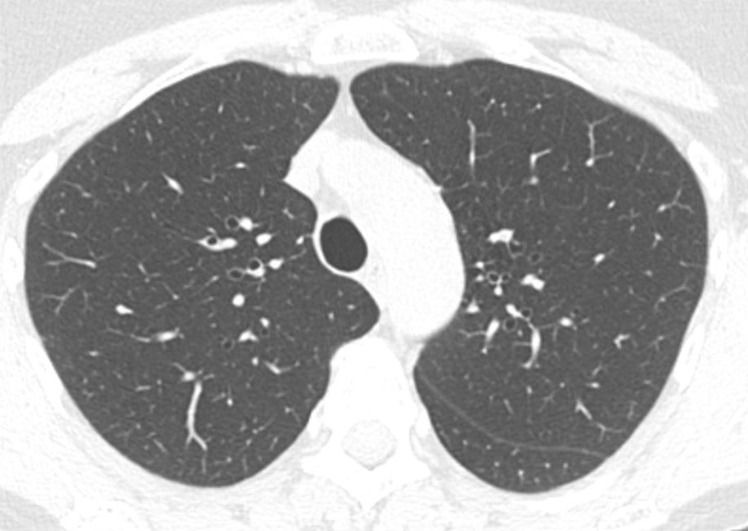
Axial thin-section CT scans in a healthy patient. (a) Inspiratory examination reconstructed with 5-mm thick sections and soft-tissue reconstruction demonstrates relative loss of definition of the interlobular septa and secondary pulmonary lobule. (b) Inspiratory sequence reconstructed with 1-mm thin sections and moderate edge-enhancing kernel reconstruction shows normal appearance. (c) Expiratory imaging shows bowing of the posterior tracheal wall and diffuse mildly heterogeneous increase in lung attenuation. Note that mild heterogeneous lung attenuation is normal.
